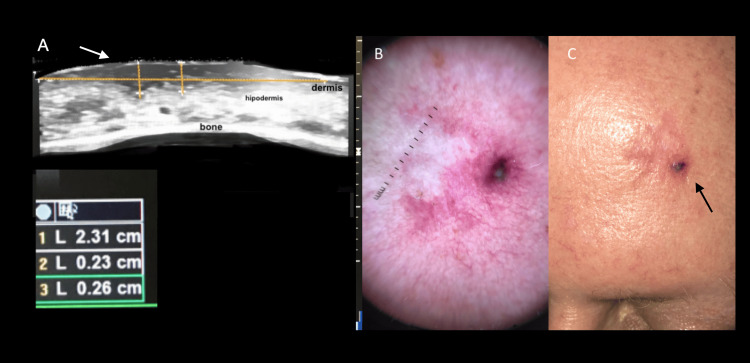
Ultrasound, dermoscopy, and clinical features of basal cell carcinoma. (A) High-resolution ultrasound shows a well-defined, hypoechoic, oval subepidermal lesion (white arrow). It involves the entire dermis and contains hyperechogenic punctiform areas. The horizontal yellow line shows the transverse diameter of the lesion; the vertical yellow lines show the tumor thickness. (B) Dermatoscopy shows a non-melanocytic lesion with arboriform telangiectasias. (C) Clinical image of the same patient (black arrow) shows the left frontal patch lesion with confirmatory biopsy of basal cell carcinoma.
